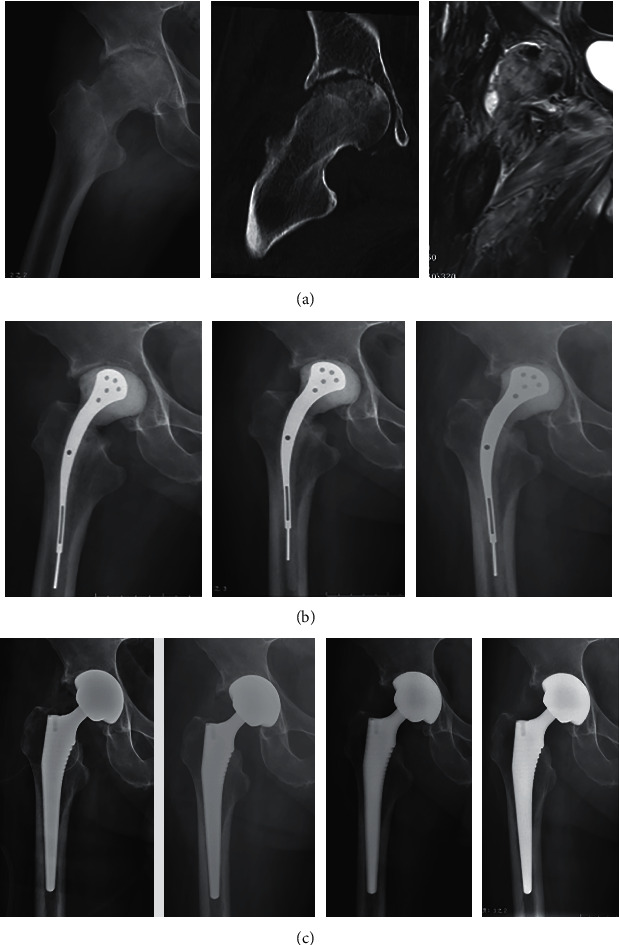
Radiographs of a 49-year-old man with pain in his right hip. (a) Anteroposterior X-ray and coronal CT images of the pelvis showed narrowing of hip joint space and destruction of bone. Coronal MRI (cut) of the pelvis showed diffuse edema of the right hip joint involving almost all muscles of the right hip. (b) The femoral head was excised and implanted with an antibiotic cement prosthesis: 5 days, 1 month, and 3 months after the operation, respectively. (c) The postoperative imaging findings of hip replacement at 0 days, 1 month, 3 months, and 19 months, respectively.
